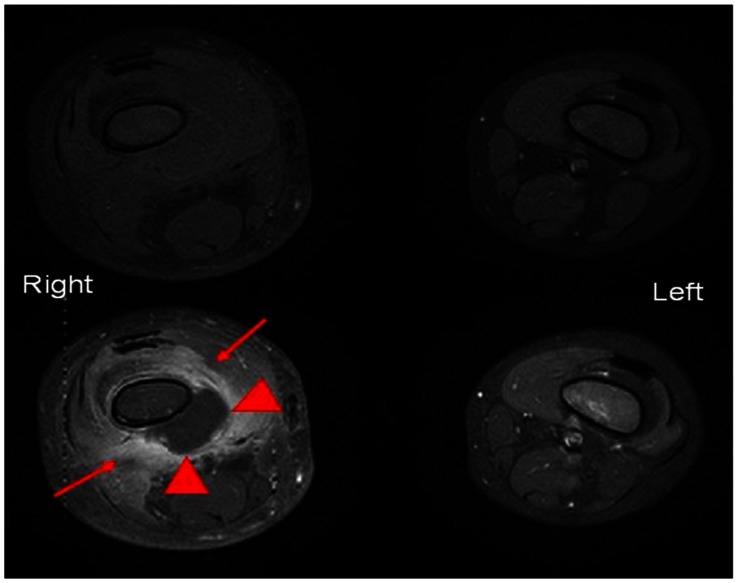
Axial MRI images through the distal femora in a 4-year-old with osteomyelitis.
